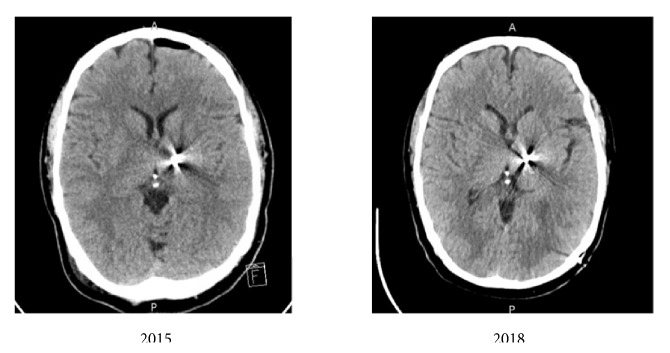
CT of head from 2015 and 2018 demonstrating no obvious movement of DBS device or pathology surrounding device.
